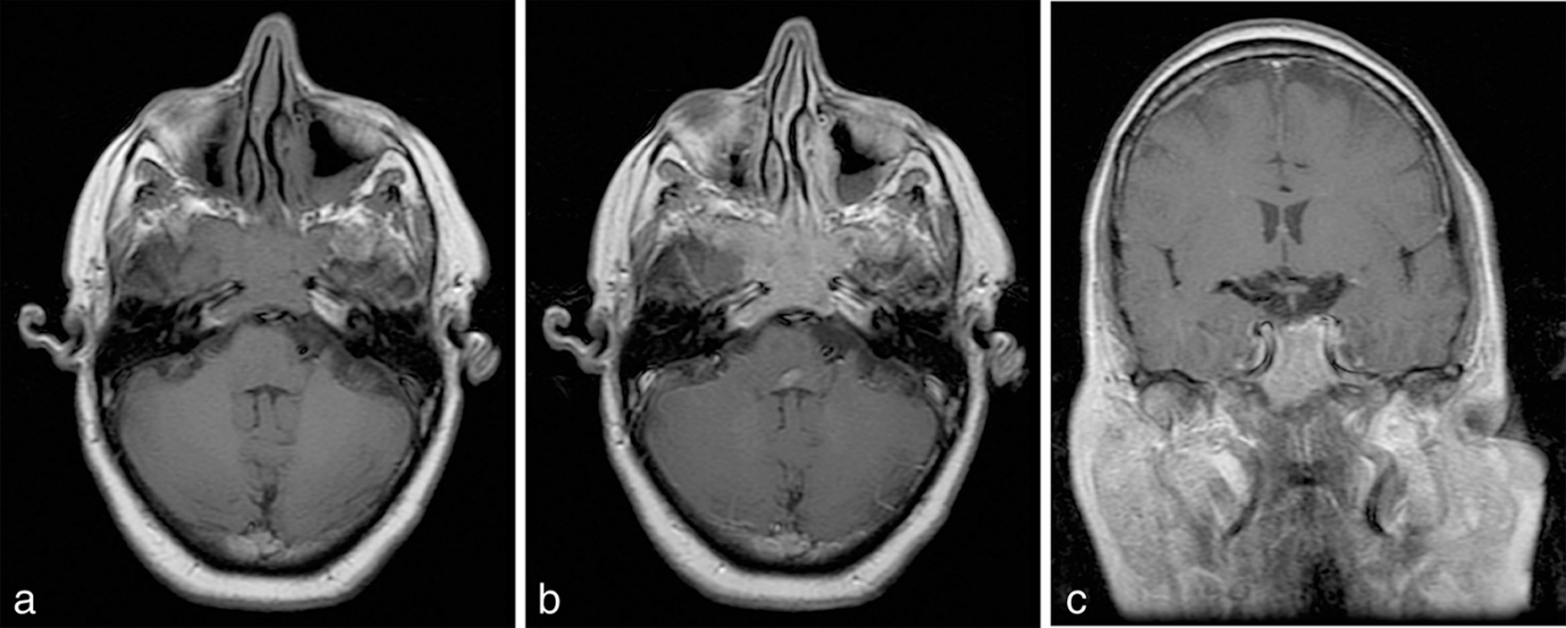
MRI scan demonstrating a mass in bilateral sphenoid sinus. (a) Axial unenhanced *T*_1_, (b) axial post-contrast *T*_1_ and (c) coronal post-contrast *T*_1_ images.
